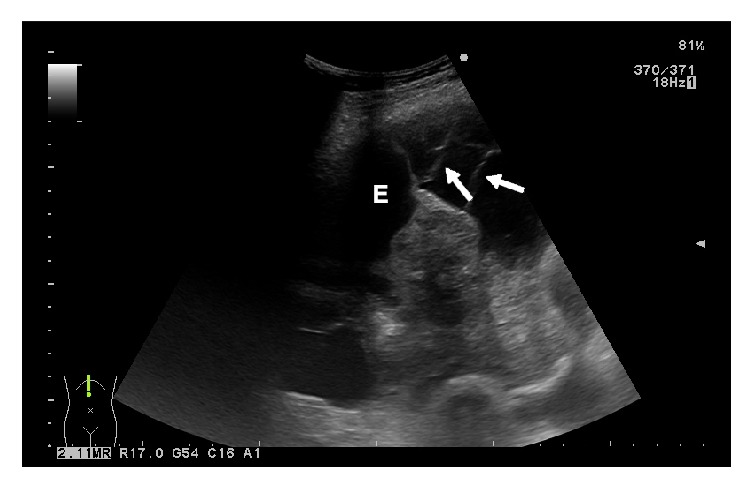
Typical ultrasonographic image of malignant pleural effusion with fibrinous septation. Fibrin strands within a pleural effusion can be seen (white arrows); E = pleural effusion.
